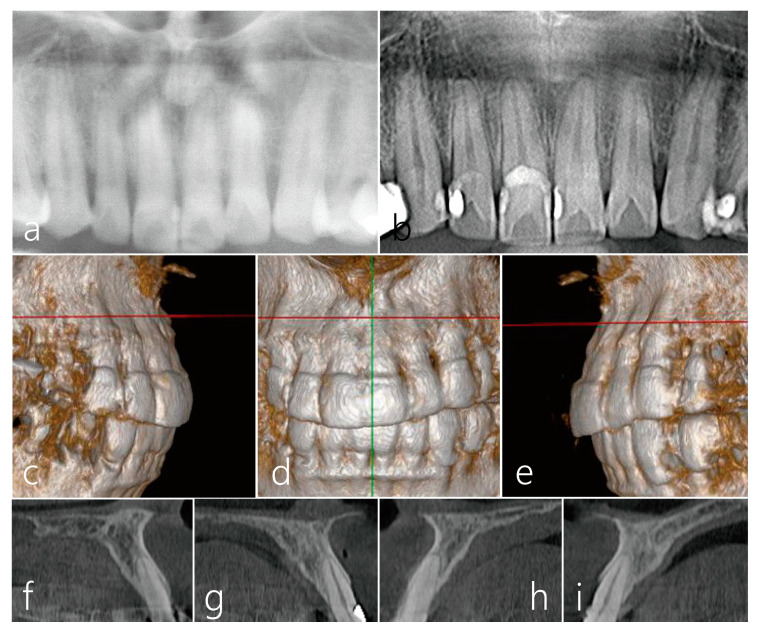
(a) A close-up image of panoramic radiograph at the 10-year follow-up. (b) Minimal radiographic bone change was observed in the maxillary anterior teeth on the close-up image of panoramic radiograph obtained 34 years after the procedure. (c–e) The 3-dimensional CBCT images taken 34 years after the procedure revealed well-maintained labial bone plates of the maxillary anterior teeth. (f–i) On the cross-sectional images of the CBCT at the 34-year follow-up, the labial bone plates of the maxillary anterior teeth were well-maintained.
